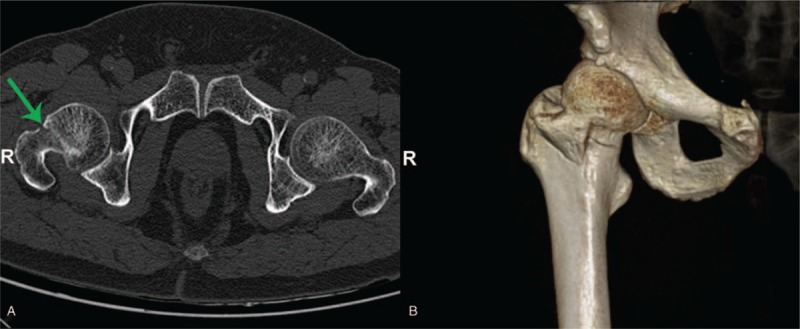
Preoperative CT of the right hip was obtained. (A) Axial plane image of right hip showed that the right femoral neck bone was discontinuous and linear translucent (green arrow), (B) three dimensional CT image of the injured right hip. CT = computed tomography.
